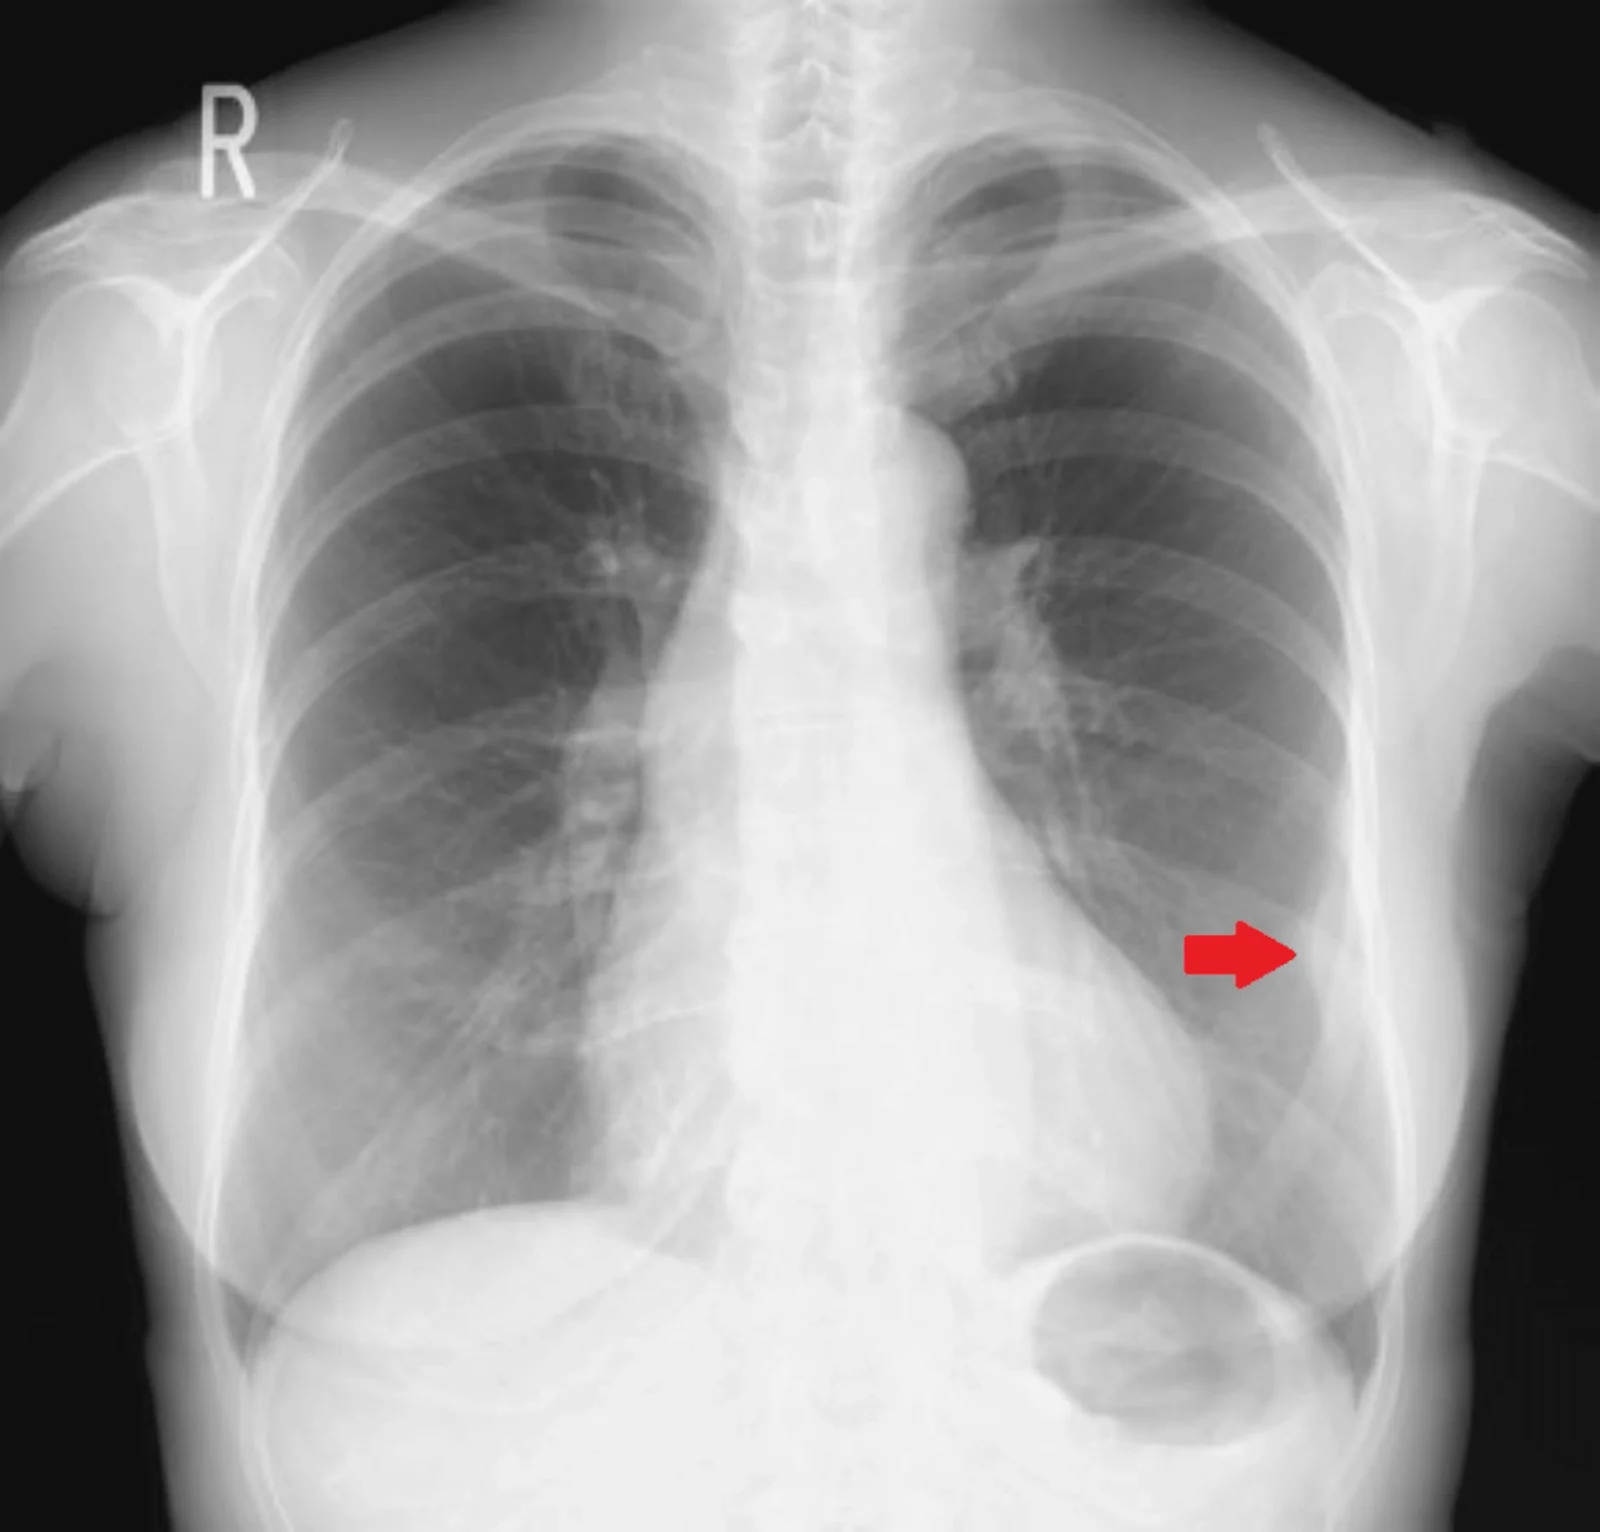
Chest radiograph Chest radiograph demonstrating a nodular shadow (red arrow) projecting over the left lower lung field along the inner border of the left seventh rib.
